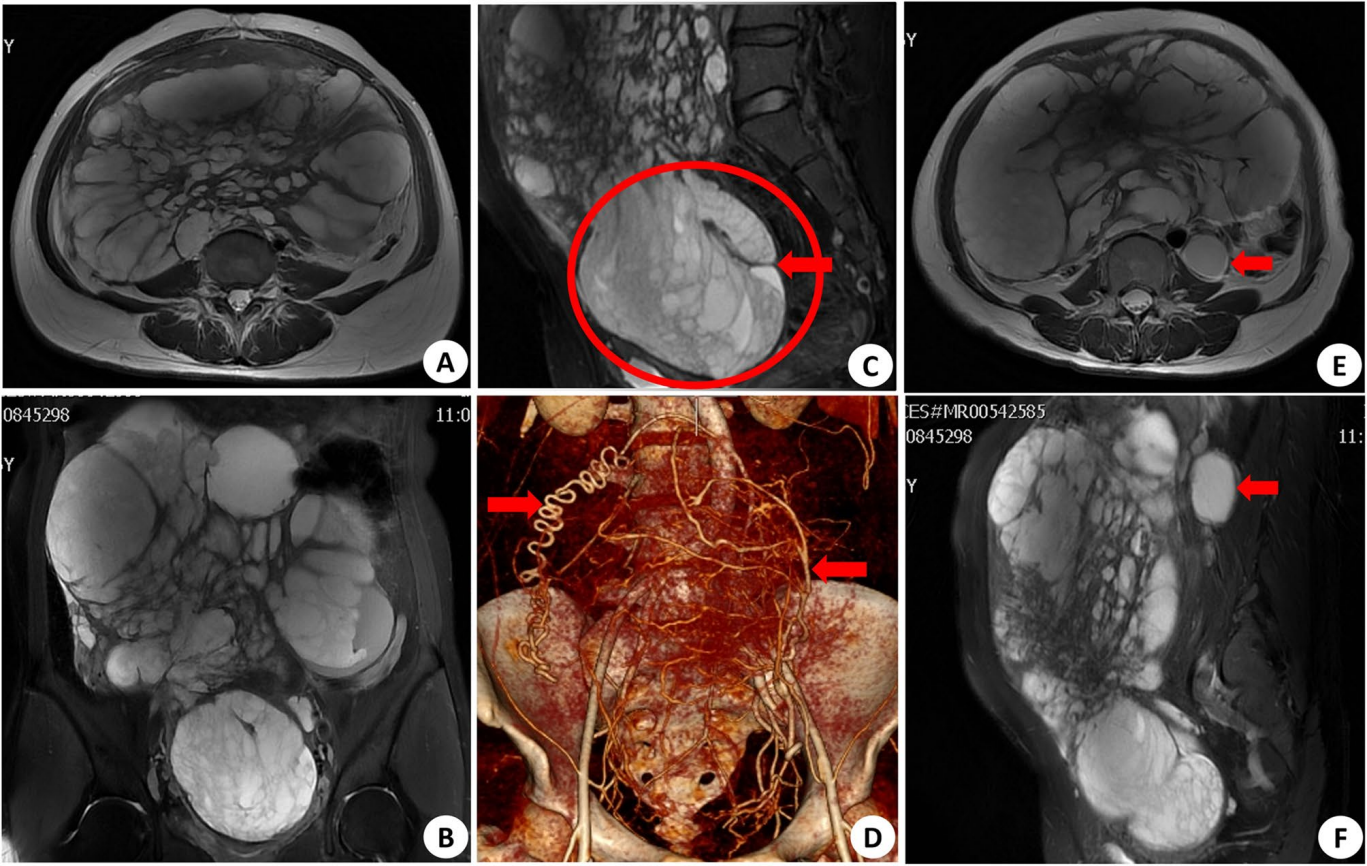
Imaging manifestations of the patient. Axial (A), coronal (B) and sagittal (C) nonenhanced MRI of the pelvis demonstrates enlarged uterus, the circle and the arrow show distorted cervix and an open external cervical opening and vaginal vault. CT three-dimensional reconstruction (D) showed abundant blood supply of the uterus (arrow). Axial (E) and sagittal (F) MRI of the lymph nodes in the left retroperitoneum of aorta (arrow)
